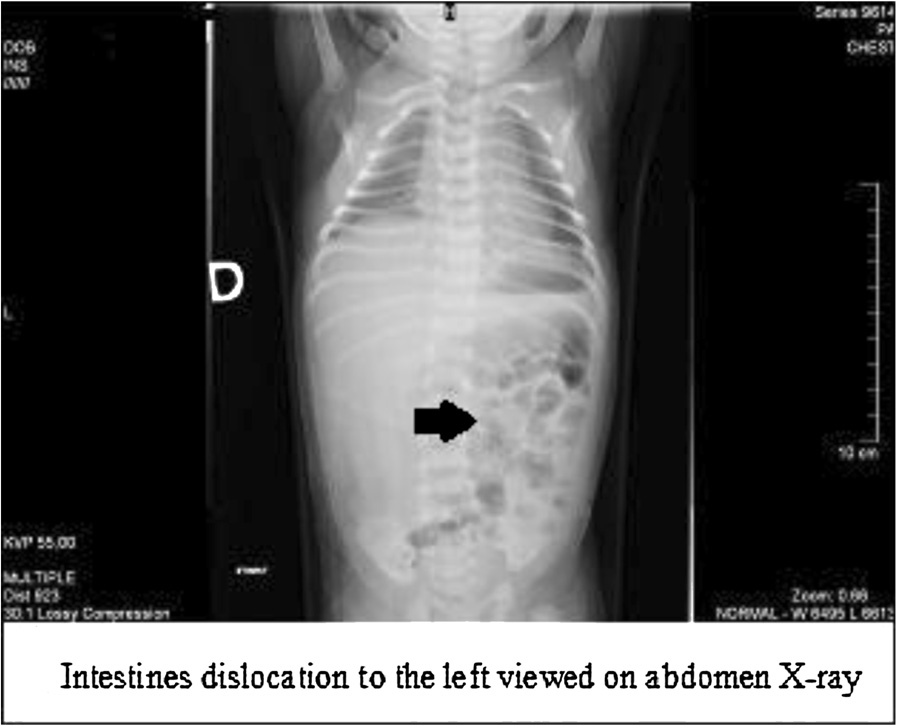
Dislocation of the intestines to the left (arrow) viewed on abdomen X-ray.
